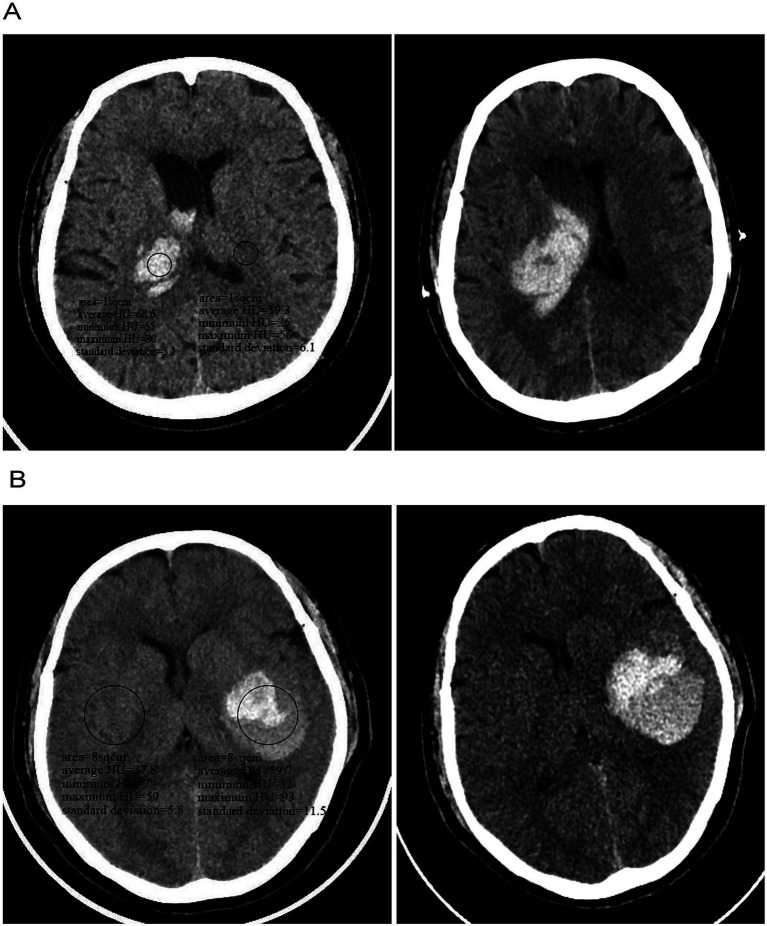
Two cases of patients with hemorrhage. (A) A 70-years-old female case with right thalamic hemorrhage. The onset time was 14:38 on Sep 1, and the time of arrival at the emergency department of our hospital was 17:10. The first NCCT scan was completed at 17:23. The CT attenuation of the largest region of interest on the side of the hematoma was 68.6 HU, and the CT attenuation of the area of greatest interest contralateral to the hematoma was 39.3HU. So, the relative CT attenuation value was 29.3 HU. Four hours later, the follow-up NCCT indicates the hematoma had expanded to 15.7 mL. (B) A 47-years-old male case with left basal ganglia cerebral hemorrhage. The onset time was 15:28 on Nov 29, and the time of arrival at the emergency department of our hospital was 18:00. The first NCCT scan was completed at 18:08. The CT attenuation of the largest region of interest on the side of the hematoma was 59.7 HU, and the CT attenuation of the area of greatest interest contralateral to the hematoma was 37.8 HU. The relative CT attenuation value was 21.9 HU. Twenty hours later, the follow-up NCCT indicates the hematoma had expanded to 44.2 mL. NCCT, non-enhanced computed tomography; HU, Hounsfield units.
